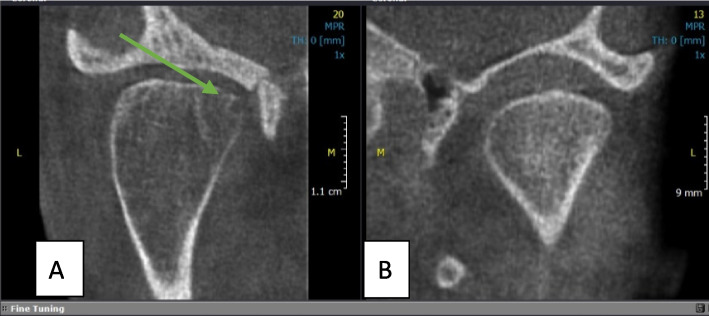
Coronal CBCT view of two patients with Condylar Hperplasia (A and B). Note the erosion in the medial pole of the Condyle (arrow in A)
